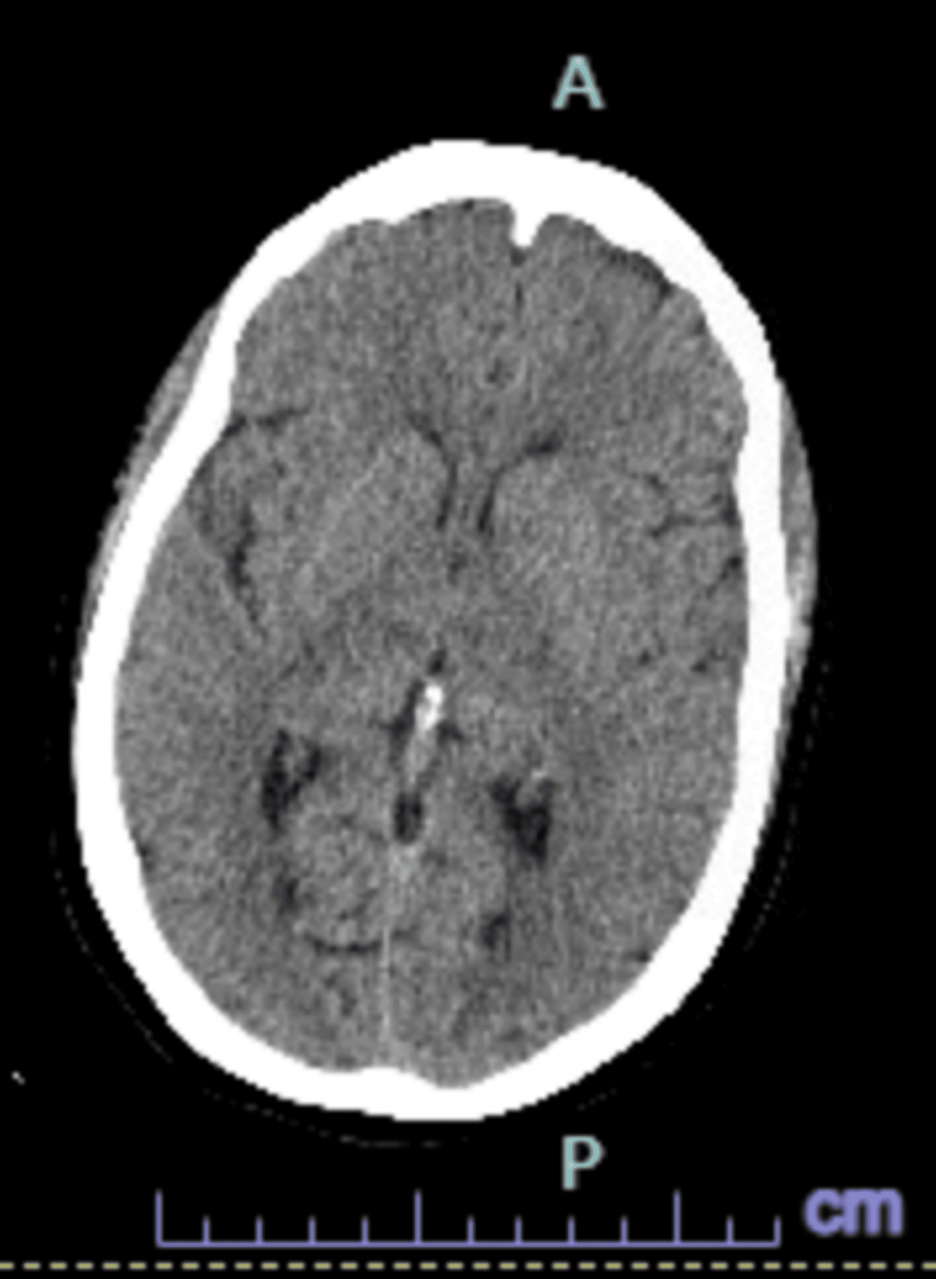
CT Code Stroke Head Without Contrast. Axial View. Taken at the time of the Code Stroke. This was negative for acute infarct, hemorrhage, or midline shift, demonstrating why CT, while abundantly used, is not a good method to detect early infarction. CT: computed tomography
